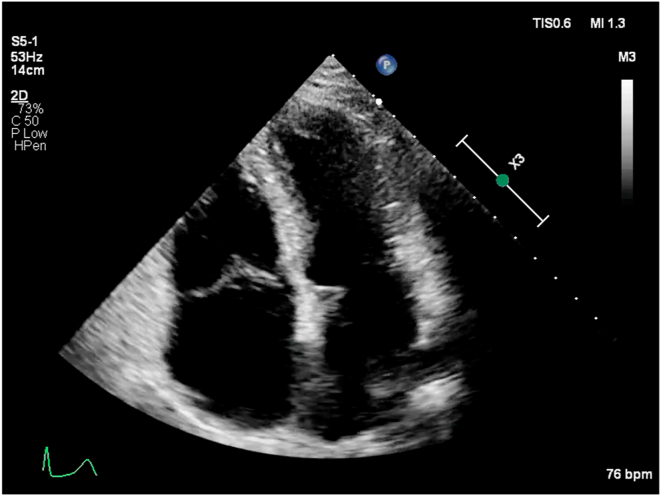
Two-dimensional TTE, apical 4-chamber systolic view, demonstrates a dilated RV and RA with abnormal septal position consistent with right ventricular volume overload.
